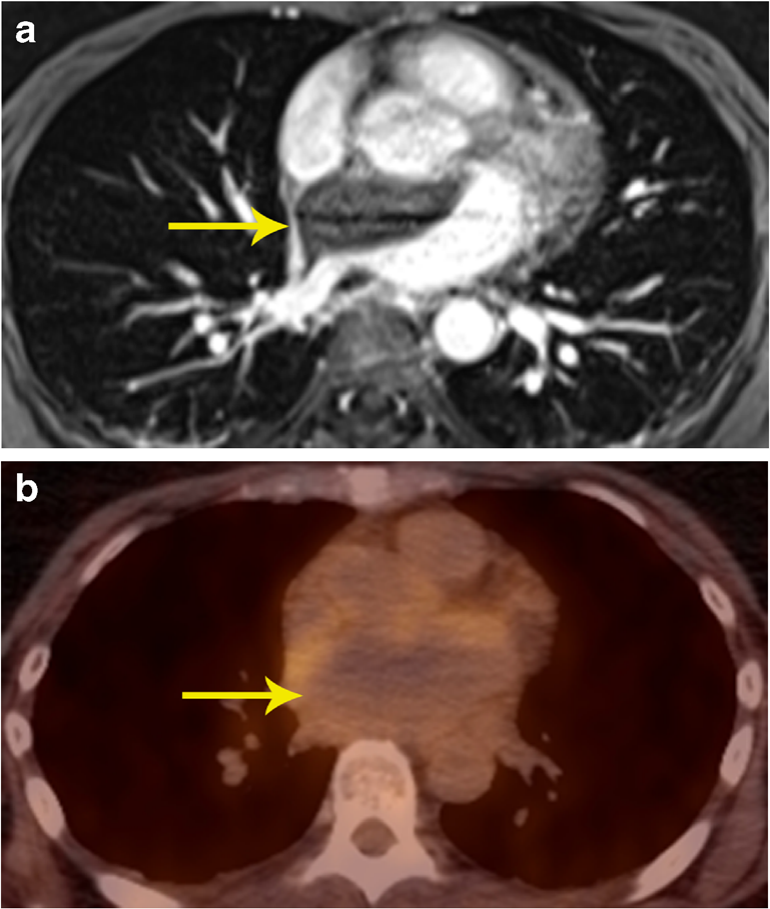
Characterization of mass. a Contrast-enhanced 3d SSFP MR image in a patient who presented with acute onset chest and back pain, shows a non-enhancing mass located in the atrial septum and left atrium extending to the mediastinum. b FDG-PET shows that there is no significant tracer accumulation in the mass. Based on the imaging findings, a neoplasm was excluded and a diagnosis of atrial hematoma was made. Follow-up imaging (not shown here) showed improvement
